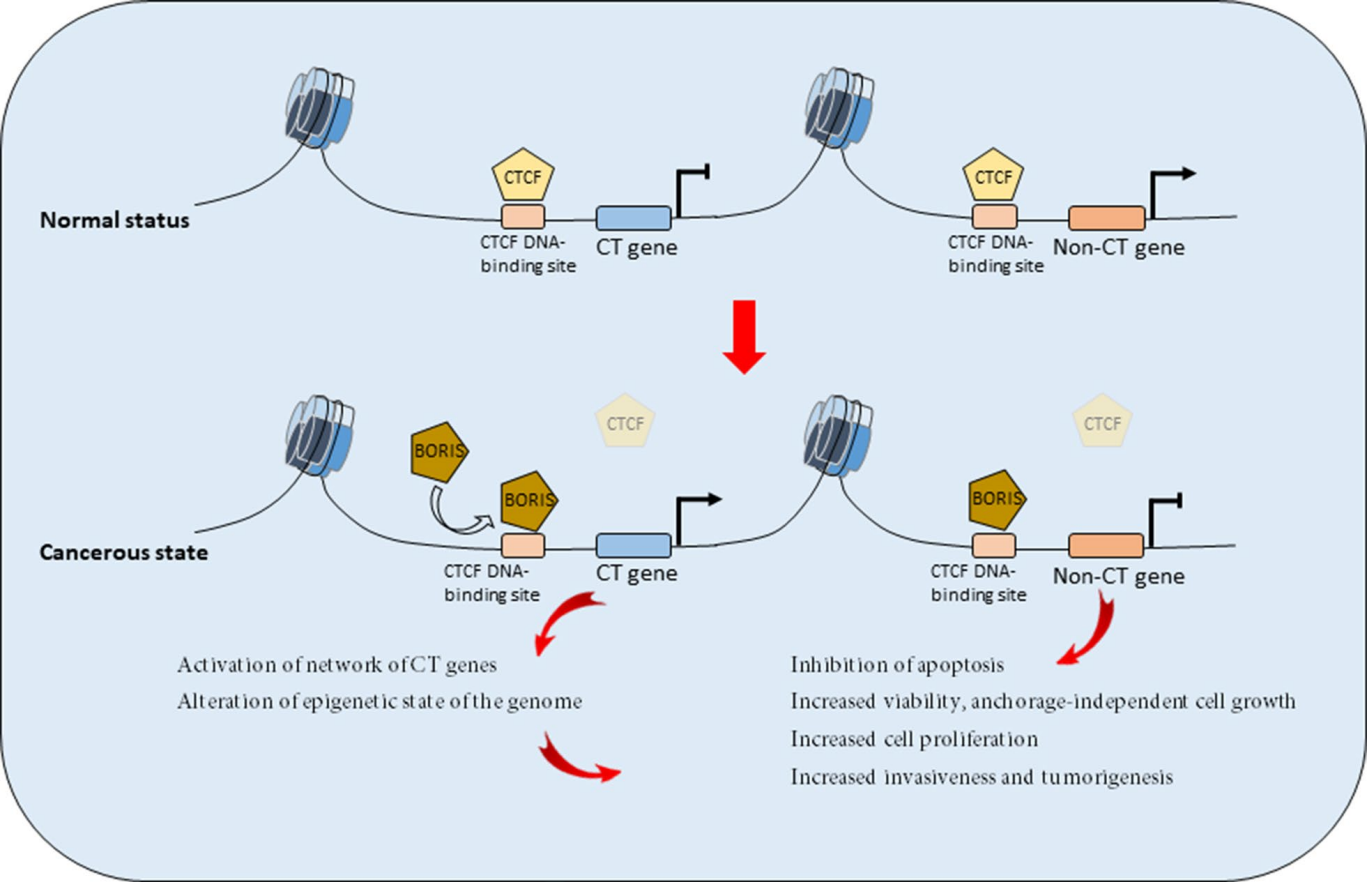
Role of BORIS in tumorigenesis. Increased expression of BORIS, shifts the competition between CTCF and BORIS for binding to CTCF DNA-binding site in favor of BORIS. This leads to the replacement of CTCF by BORIS at promoters of some cancer-testis (CT) genes including MAGE-A1, MAGE-A2, MAGE-A3, MAGE-A4, MAGE-B1, MAGE-B4, GAGE-3-8, RAGE-2, NY-ESO-1 (CTAG1B), LAGE-1 (CTAG2), FerT and TSP50, and some non-CT genes such as BRCA1, Oct-3/4 (POU5F1), MYC, Rb2/p130, SBSN, and hTERT, and androgen, progesterone and estrogen receptors. Expression of target genes leads to cancer progression via activation of the network of CT genes, inhibition of apoptosis, induced cell growth, and increased proliferation and invasiveness of cancer cells
